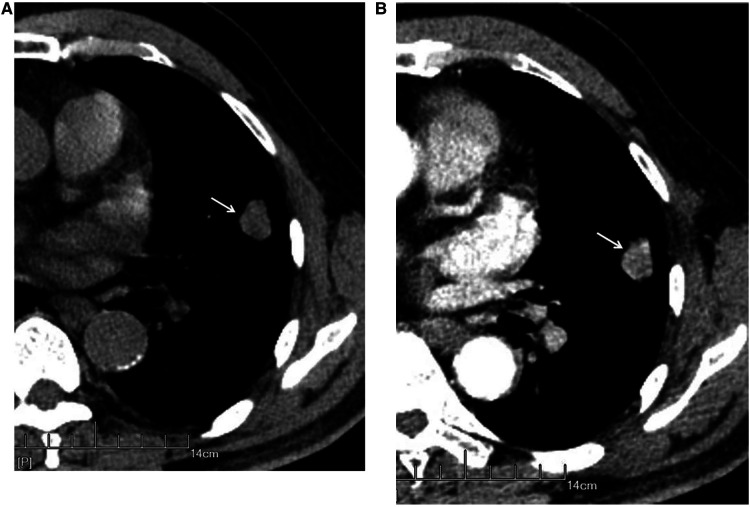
Male, 69 years old, with infiltrating adenocarcinoma in the anterior segment of the upper left lung (moderately differentiated degree) without hilar and mediastinal lymph node metastases. CT showed a solid nodule. The left figure (A) shows unenhanced imaging and a solid nodule (arrow), and the right figure (B) shows enhanced imaging and an enhanced nodule (arrow).
